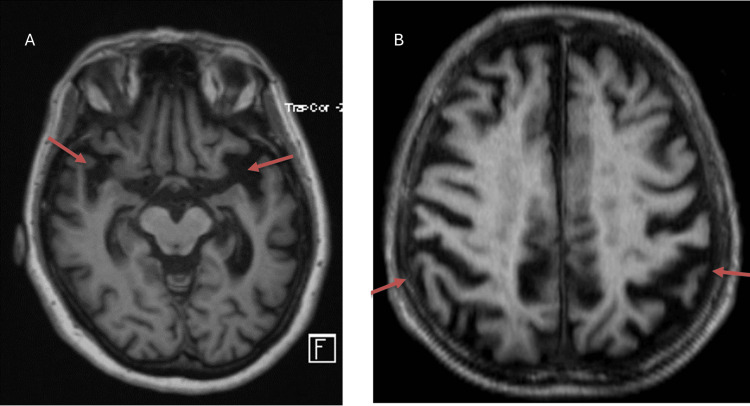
T1-weighted MRI axial images (A,B) showing cortical atrophy in the temporal and parietal regions (arrows) MRI: magnetic resonance imaging
